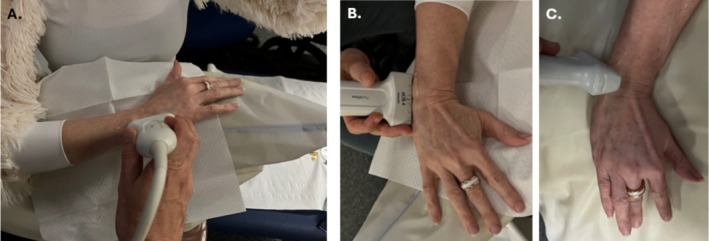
Positioning of the wrist for sonographic assessment of the ulnar aspect. (A) Patient is seated, hand pronated, forearm elevated to shoulder height, with a pillow supporting the forearm allowing long axis imaging from a dorso‐ulnar approach. (B) Long axis imaging with the hand radially deviated to open the ulno‐carpal space. (C) Short axis imaging with the hand radially deviated.
